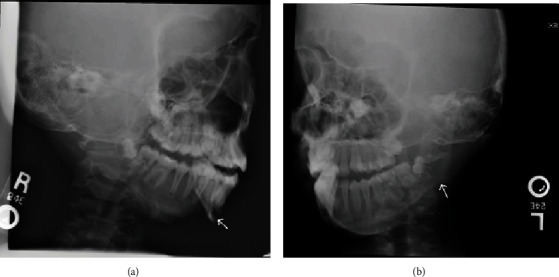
Mandibular X-rays on hospital day 2 displayed a lytic lesion with cortical breakthrough adjacent to the mandibular symphysis and a small area of erosion at the mandibular angle.
